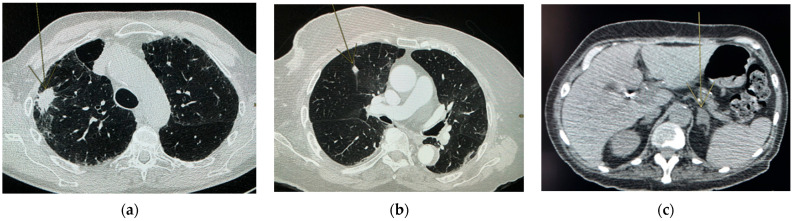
Suspicious mass and nodule. (a) Chest CT of an 81-year-old man performed in the context of suspected COVID-19 pneumonia with de novo discovery of suspicious mass. After biposing the lesion, the diagnosis is aspergilloma with usual interstitial pneumonia. Suspicious mass (> 3 cm) in the right upper lobe. (b) Chest CT of an 88-year-old woman performed in the context of suspected COVID-19 pneumonia, abdominal pain, nausea and vomiting with de novo discovery of suspicious nodule. Left lower lobe subpleural nodule. (c) Chest CT of a 62-year-old woman performed in the context of suspected COVID-19 pneumonia with de novo adrenal incidentaloma.
